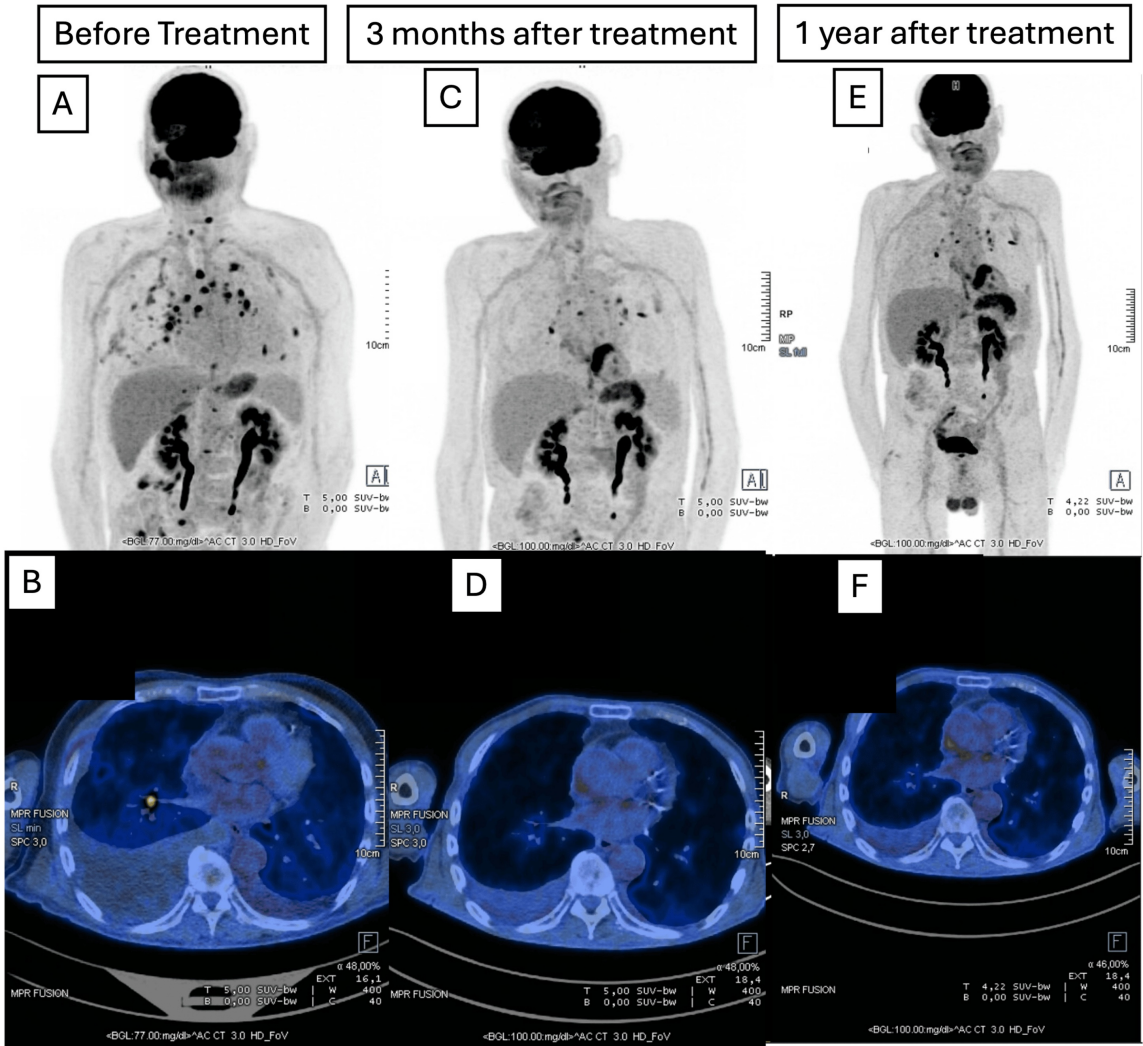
PET-CT scan comparative results: before treatment (A,B), three months (C,D), and one year (E,F) after the initiation of treatment. PET-CT: Positron Emission Tomography-Computed Tomography; AUC: Area Under the Curve.
